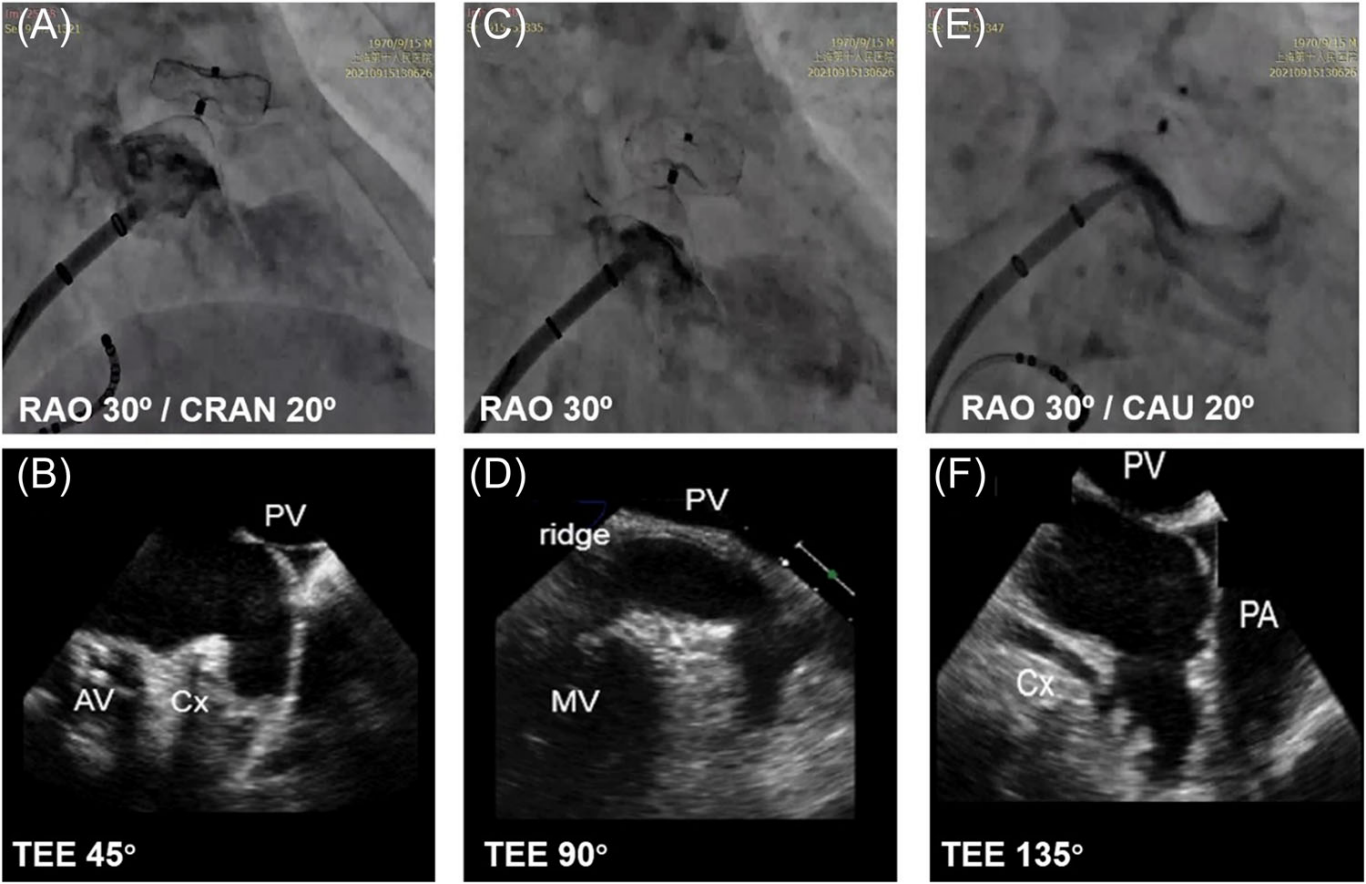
The routine views of digital subtraction angiography and TEE for evaluation of the combined procedure. (A, B): LAA angiography at RAO 30°/CARN 20°corrected with TEE 45°; (C, D): LAA angiography at RAO 30° corrected with TEE 90°; (E, F): LAA angiography at RAO 30°/CAU 20° corrected with TEE 135°. CAU, caudal; CRAN, cranial; RAO, right anterior oblique; TEE, transesophageal echocardiography.
